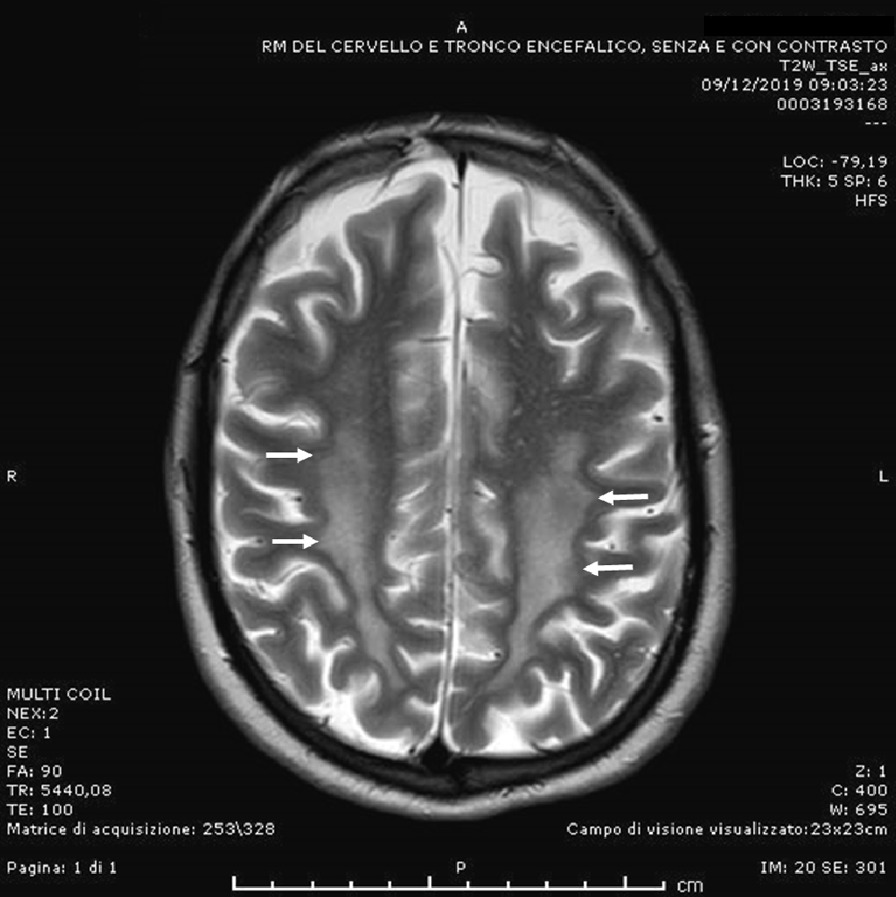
Brain MRI. In the T2-weighted sequences, foci of altered hyperintense signal of the bilateral fronto-temporo-parieto-occipital subcortical white matter are evident (white arrows)
